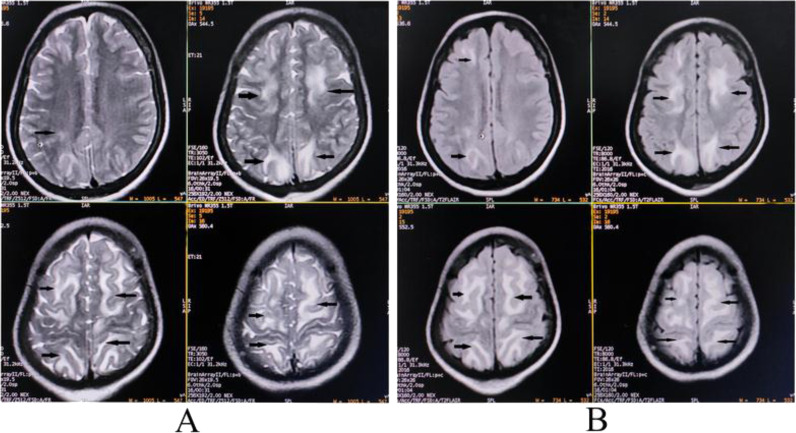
MRI of the brain revealing hyperintensity on tbl2-weighted (A) and tbl2-FLAIR, (B) images involving bilateral frontal and parieto-occipital grey-white interface (arrows), suggestive of PRES.
